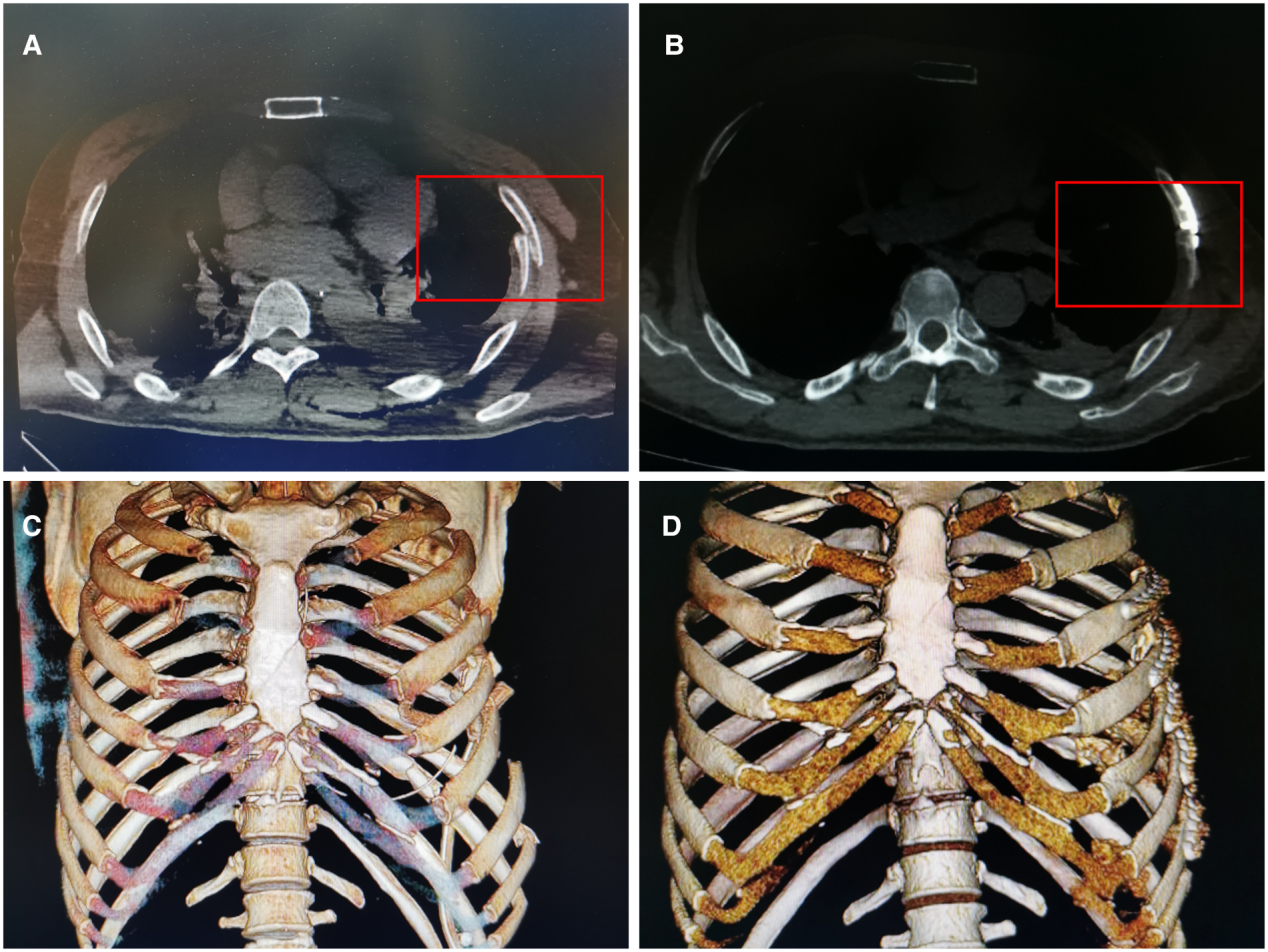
CT scan images of a patient with rib fracture. The same patient as Figure 1. (A) Preoperative CT scan suggested a left rib fracture. Inside the red box is the broken ends. (B) Three months after surgery, CT axial plain scan at the same level showed that the left rib fracture healed well, there was continuous callus formation at the fracture in the red box. (C) Preoperative CT 3D reconstruction suggested multiple fractures ribs. (D) Postoperative CT 3D reconstruction suggested good repositioning of the broken ends and the internal fixation.
